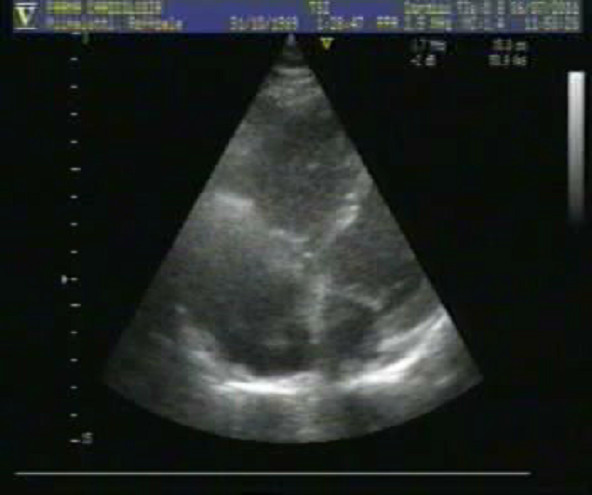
Transthoracic echocardiogram (TTE 4-chamber). Right sections were dilated with a hypokinetic right ventricle. An image referring to a thrombosis was detected in the rigtht atrium.
